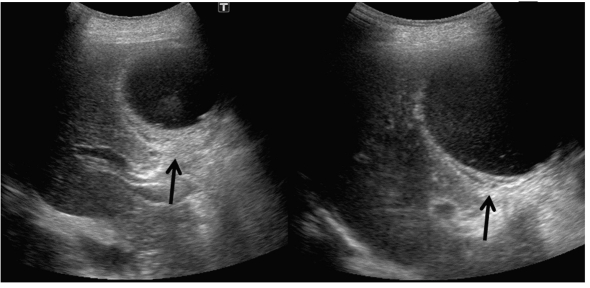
Abdominal ultrasound showed enlarged and distended gallbladder containing debris, and thickness of the wall of gallbladder.
